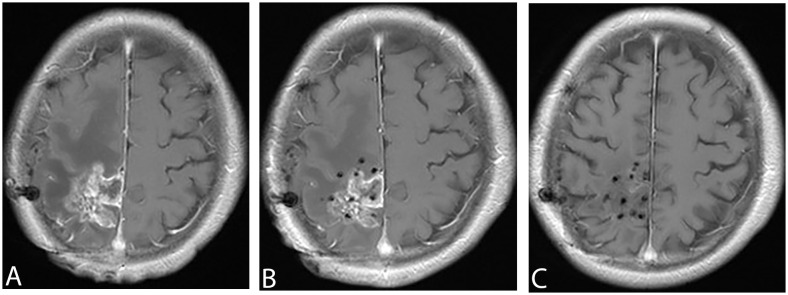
Symptomatic brain edema after ^125^I seed implantation in a patient with recurrent glioblastoma. (A) Preoperative contrast-enhanced MRI showing the tumor in the right parietal lobe with surrounding peritumoral edema. (B) One week after ¹²^5^I seed implantation, the patient developed headache and left hemiparesis. MRI confirmed postoperative brain edema. One-week treatment with mannitol and corticosteroids resulted in significant symptomatic relief. (C) There-month follow-up MRI showed marked reduction in tumor enhancement and significant alleviation of brain edema compared with baseline.
